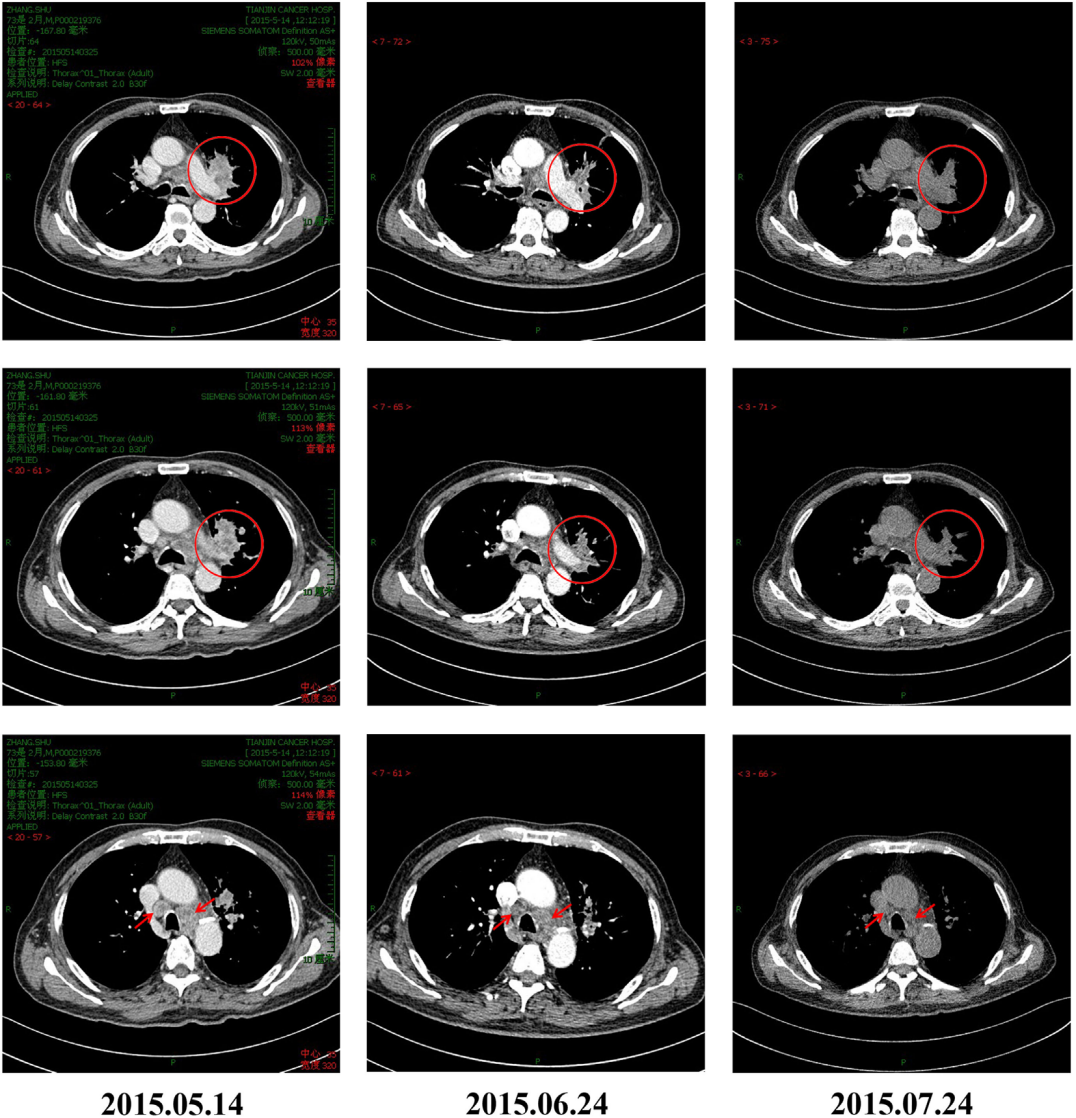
CT scans of the patient before and after pembrolizumab and CIK combination treatment for advanced NSCLC. Tumor masses can be seen in the left lung hilum and mediastinal lymph nodes of the patient before initiation of immunotherapy. The masses significantly reduced in size on CT obtained 30 and 60 days after treatment, indicating good response to combination therapy.
